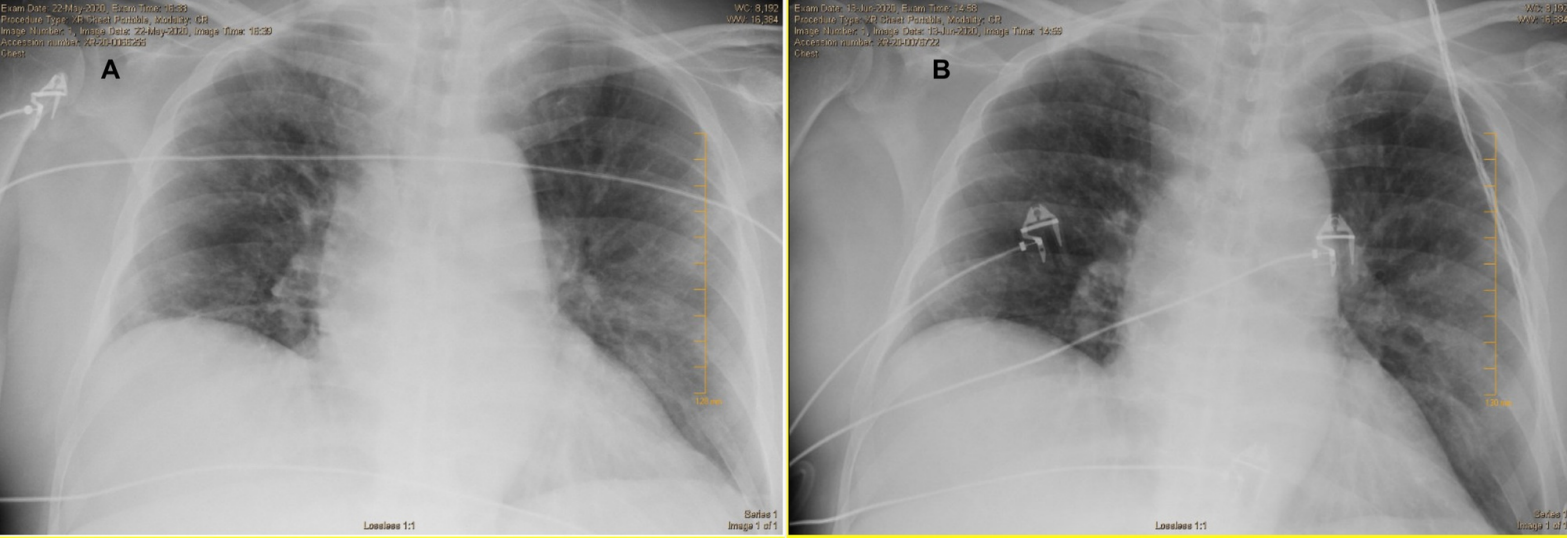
(A) Chest x-ray shows diffuse bilateral interstitial and peripheral opacities. (B) Improved bilateral patchy and hazy airspace opacities.
